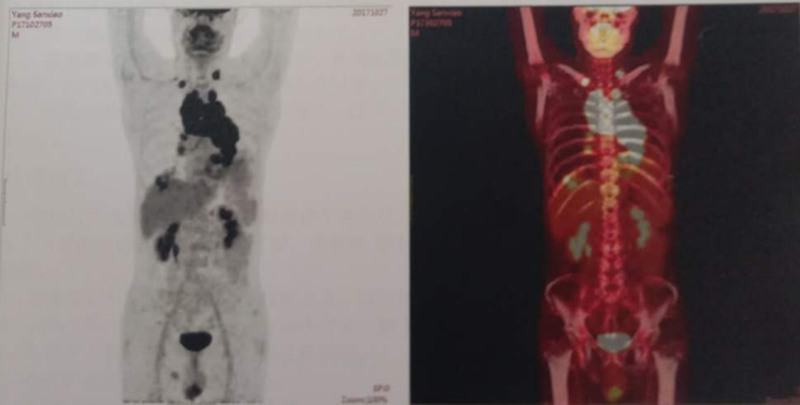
PET/CT (p17102705). PET/CT (p17102705) of Baotou Central Hospital showed hypermetabolic mass in left hilar area, which was considered to be malignant with multiple lymph node metastases in left supraclavicular region, right subclavian region, uppermost mediastinum, paratracheal, anterior vena cava, retrotracheal vena cava, paraaortic arch, aortic window, subcarina, and bilateral hilar regions, and metastatic tumor in both lungs. The left lung biopsy showed small cell carcinoma.
